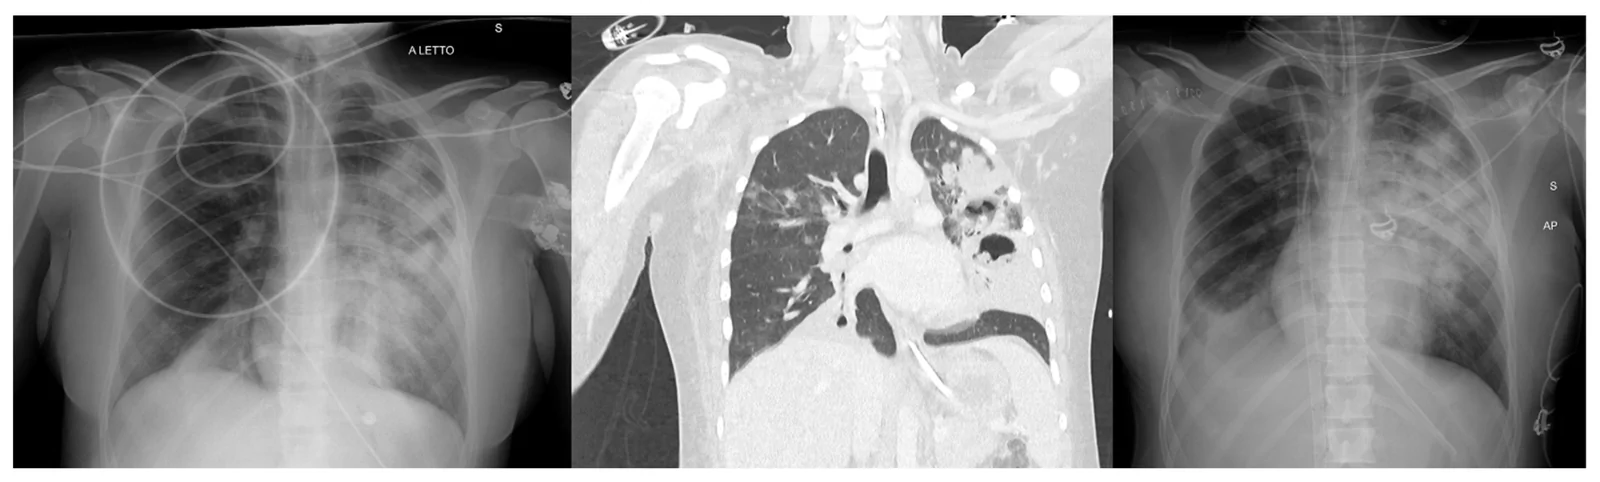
Radiological image of the case: (left) Initial chest radiograph demonstrating extensive left-sided pulmonary consolidation involving much of the left lung, consistent with pneumonia, without pleural effusion or pneumothorax; (center) coronal chest CT showing extensive left lung consolidation with cavitary change, consistent with abscess formation and overall in keeping with necrotizing pneumonia; (right) follow-up chest radiograph demonstrating an ECMO cannula projecting over the inferior vena cava in expected position; the remaining radiographic findings are otherwise unchanged.
